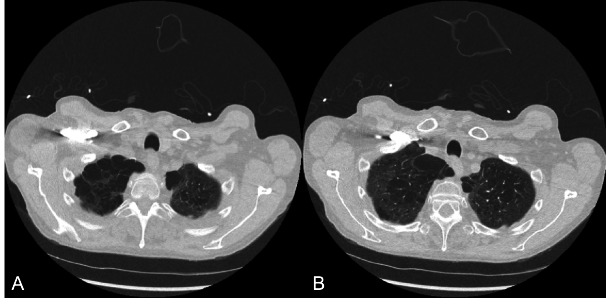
And B: Axial computed tomography images of the chest at the level of the apical subpleural blebs in the left side.
